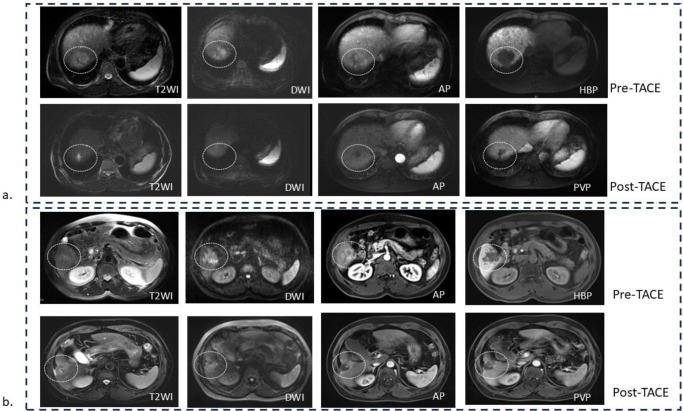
Representative examples of post-TACE response in HCC patients. (a) A 57-year-old male patient presented with a 43 mm mass in the right hepatic lobe, showing slightly hyperintense on T2WI and DWI, marked arterial enhancement, and hypointensity on the HBP. After TACE, the lesion significantly reduced in size with no residual enhancement, corresponding to OR according to mRECIST criteria. (b) A 51-year-old male patient showed a 38 mm lobulated mass with irregular margins in the right hepatic lobe, hyperintense on T2WI and DWI, exhibiting marked arterial enhancement and hypointensity on HBP. After TACE, the lesion exhibited partial necrosis, but the reduction in tumor size was less than 30%, consistent with SD. TACE, transcatheter arterial chemoembolization; HCC, hepatocellular carcinoma; T2WI, T2-weighted imaging; DWI, diffusion-weighted imaging; AP, arterial phase; PVP, portal venous phase; HBP, hepatobiliary phase; CR, complete response; SD, stable disease; mRECIST, modified Response Evaluation Criteria in Solid Tumors.
